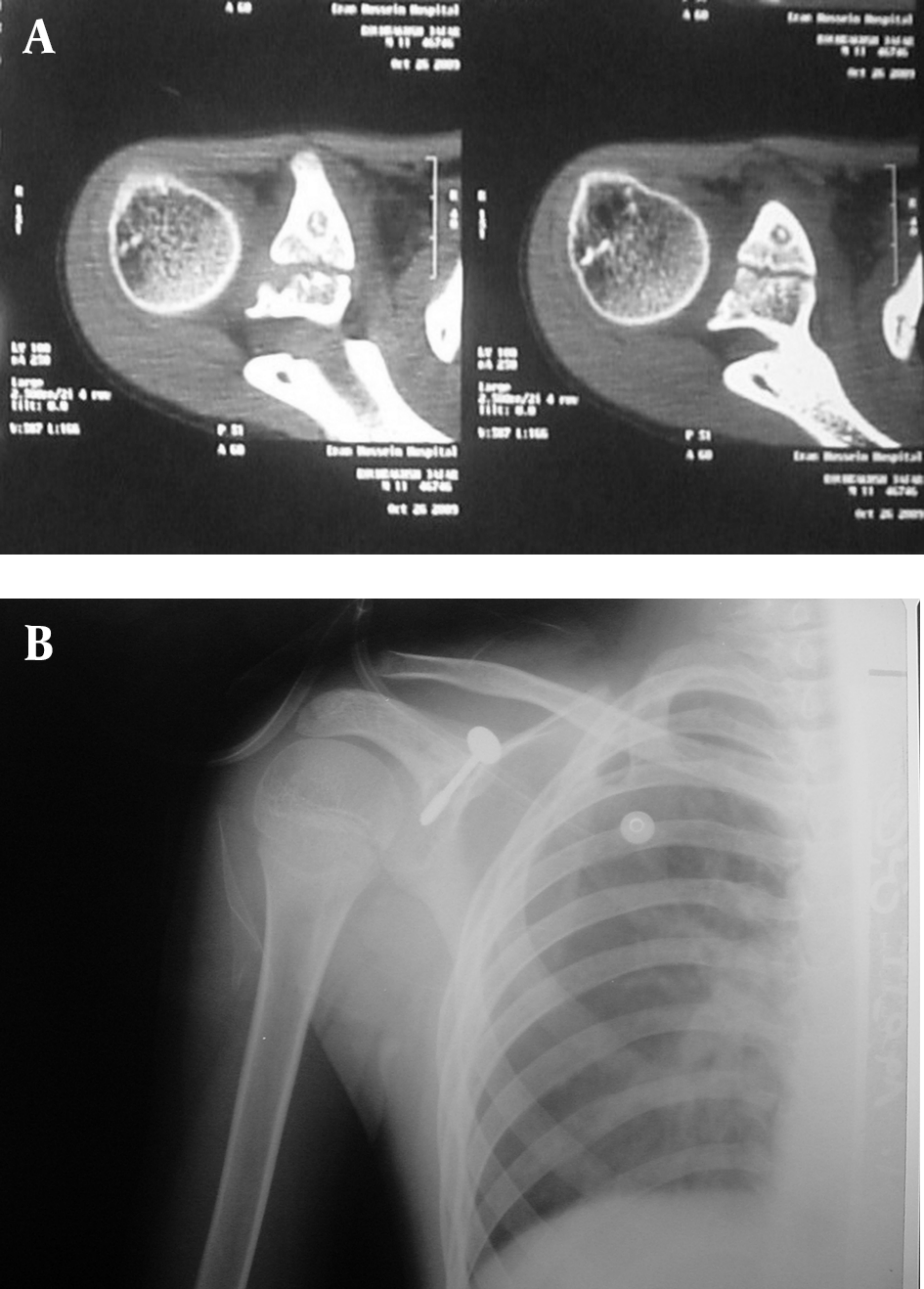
A) Apparent Nidus in Semicoronal CT-s Images, B) Screw Fixation of Coracoid After Osteotomies
